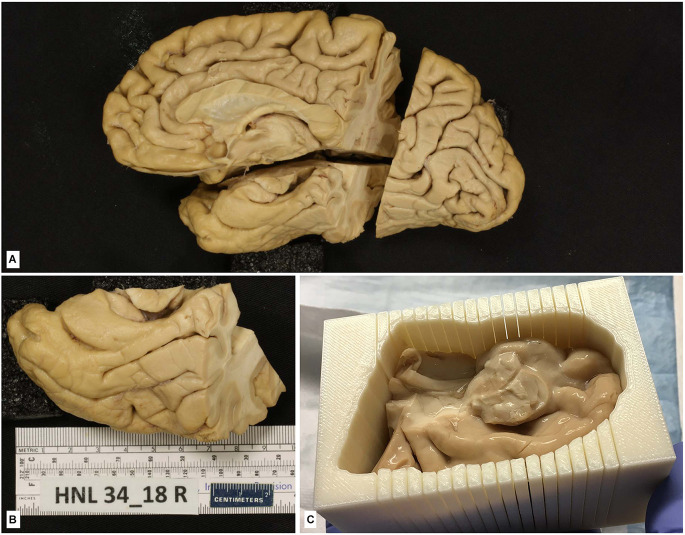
(A) The hemisphere of one of the cases of the series in Yushkevich et al. (2021). The brain hemisphere is divided into three blocks by a perpendicular cut to the *ac-pc* line extending into the temporal lobe, which is further isolated for that particular study. (B) Isolated temporal lobe piece. (C) Temporal lobe is embedded in a mold built following a process of 3D reconstruction of 7T MRI images of the temporal lobe using a 3D printer; the mold shows parallel slits in the same plane as MRI images separated by 5 mm; the plane of section of the temporal lobe is kept as following slits at 2 cm.
